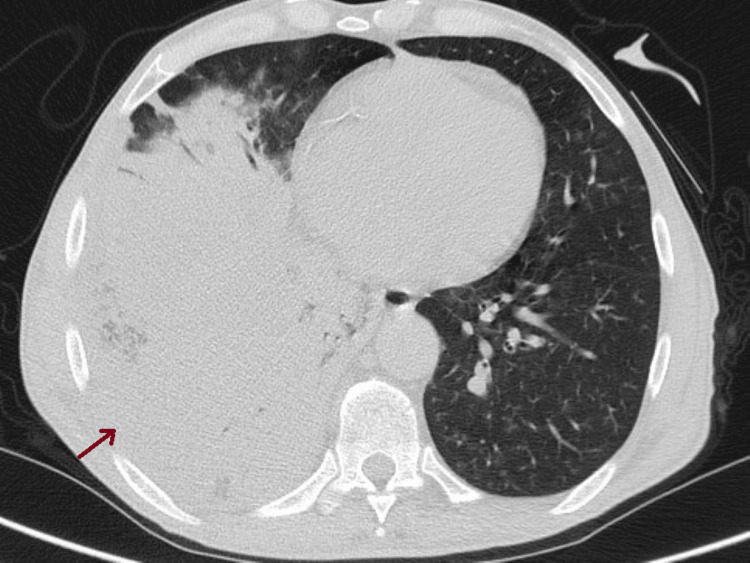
Thoracic CT scan performed upon admission shows consolidation in the right lung, with multiple nodular opacities visible (arrow).
